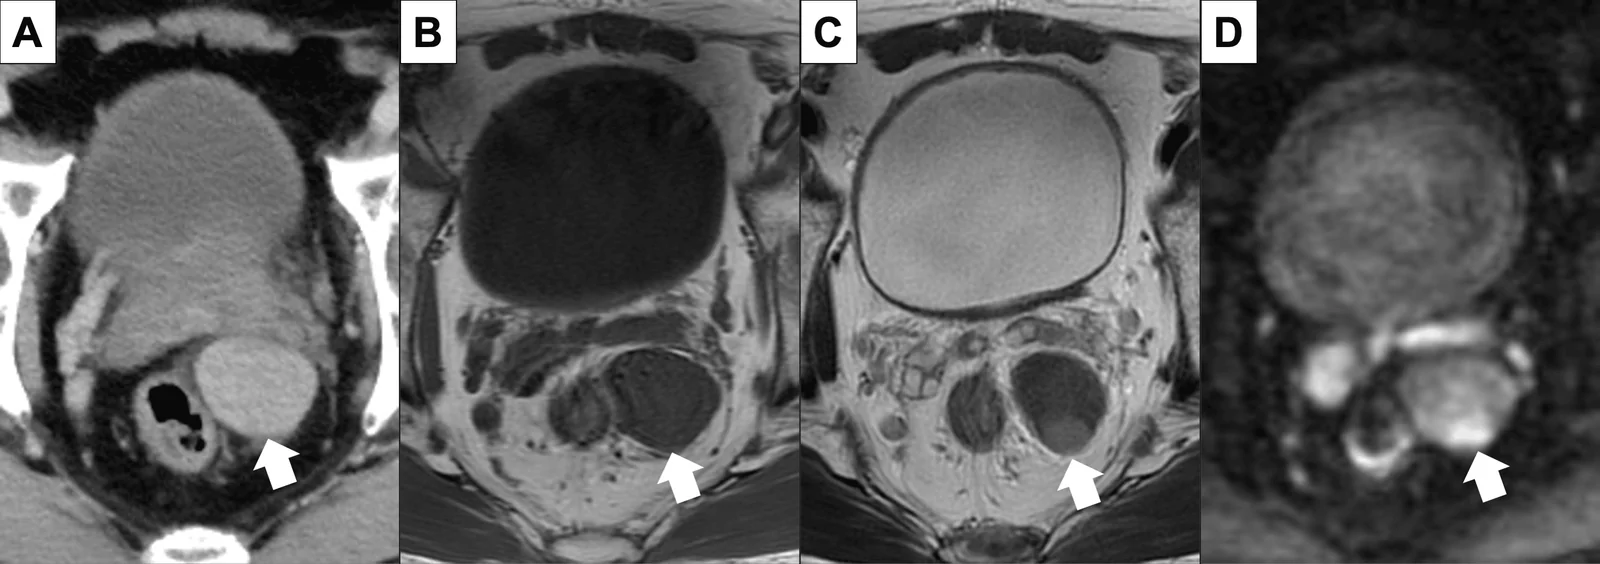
Abdominal computed tomography (CT) and magnetic resonance imaging (MRI) findings (A) Contrast-enhanced CT identifies a well-circumscribed, 35-mm tumor located between the lower rectum and the prostate. (B) T1-weighted MRI reveals a smooth-surfaced mass with low signal intensity. (C) T2-weighted MRI shows mixed low and isointense signals. (D) Diffusion-weighted imaging (DWI) exhibits isointense to focal high-signal intensity. Arrows indicate the tumor.
